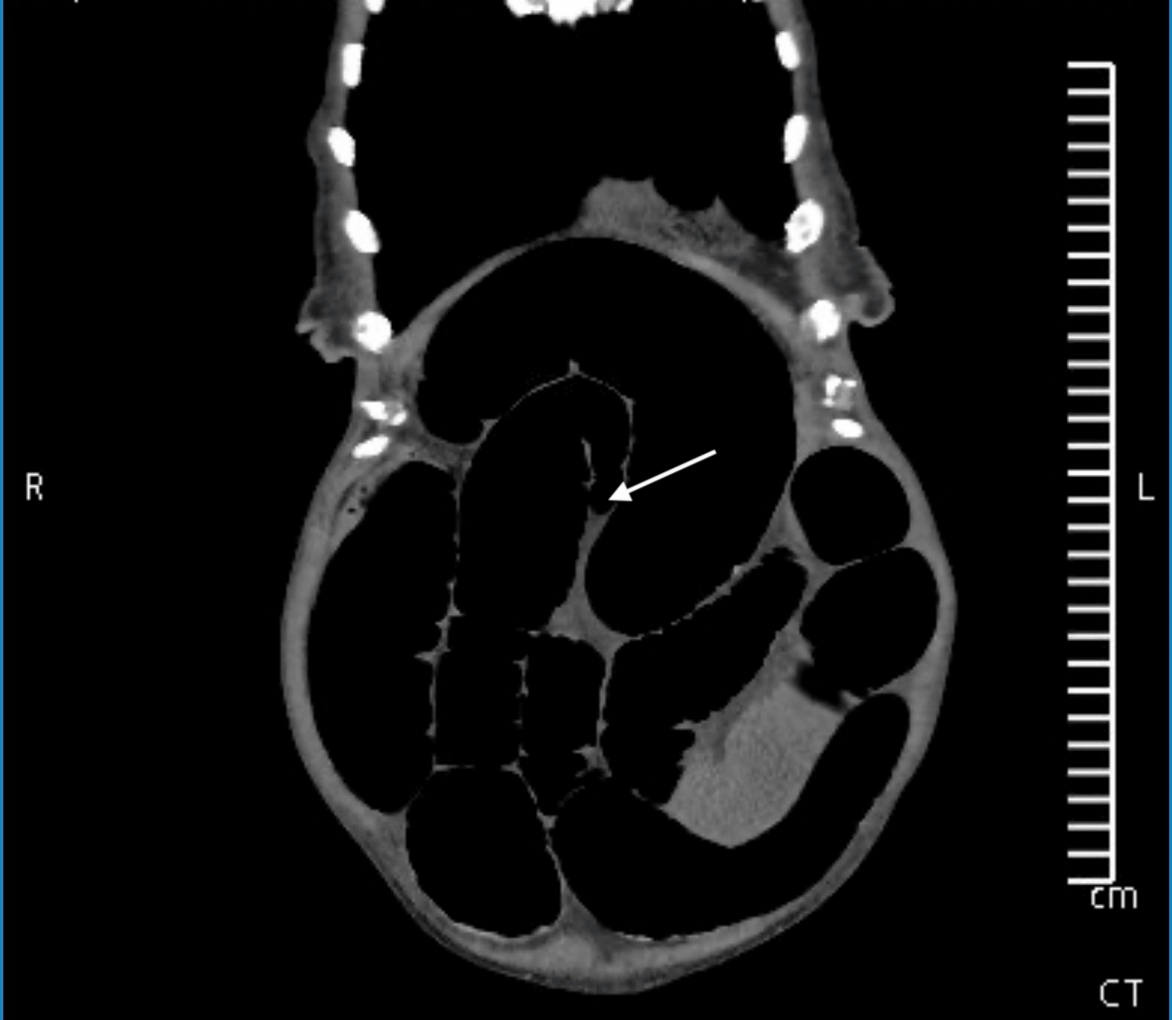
Coronal non-contrast CT image showing a small bowel transition point A non-contrast CT scan revealed dilated loops of small bowel (up to 5.6 cm) and mesenteric torsion around a transition point, indicated by an arrow. The patient's extensive small bowel diverticulosis was not visualized on imaging. This highlights that small bowel diverticulosis is often not detected radiologically, particularly in non-contrast or emergent settings, such as in this case. Small bowel diverticulosis is rare (0.3%-2.3% incidence) and is typically clinically silent. It is often identified incidentally during surgery. R: right; L: left
